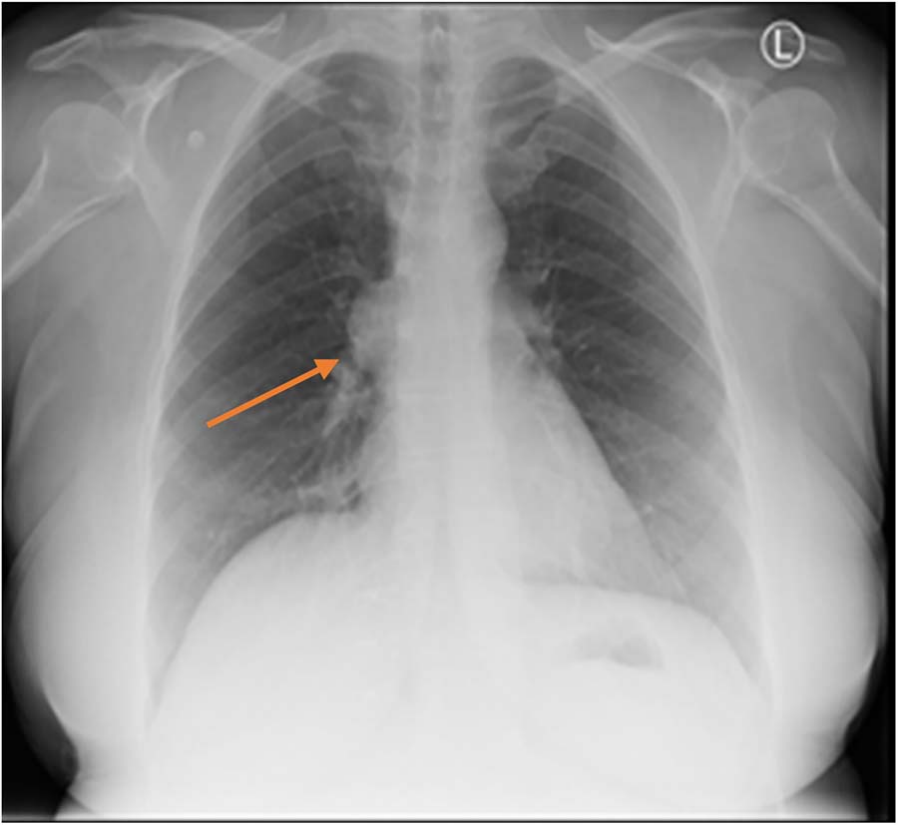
Chest X-ray showing anterior BMC (arrow).
